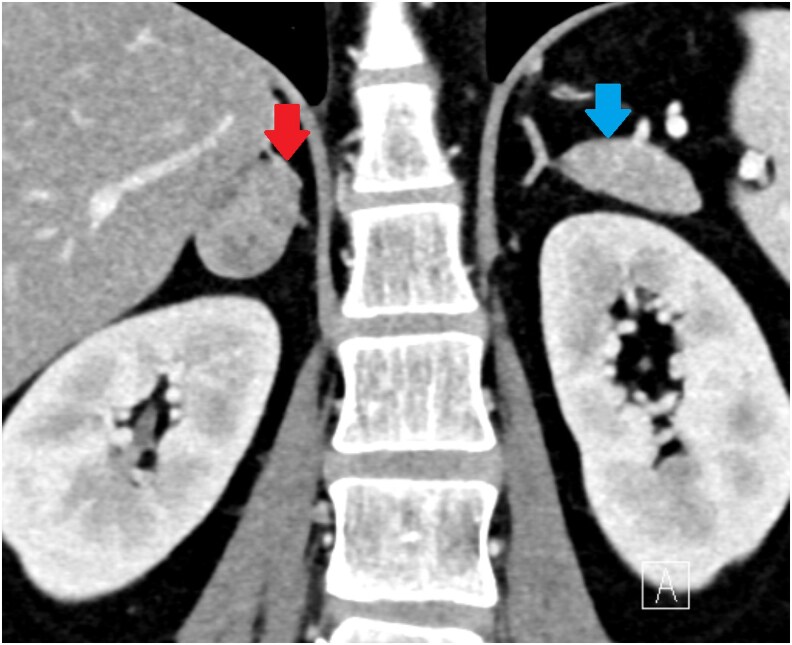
Adrenal enhanced computed tomography (CT) showed a right lipid-poor adrenal nodule (red arrow) with a diameter of 3 cm and a left lipid-poor nodule (blue arrow) with diameters of 1.1 cm (lateral limb). The right nodule was classified as indeterminate based on an absolute washout of 54% and a relative washout of 29%. In contrast, the left adrenal nodule had an unenhanced Hounsfield unit value of 54. This nodule was diagnosed as an adenoma, with an absolute washout of 78% and relative washout of 45%.
